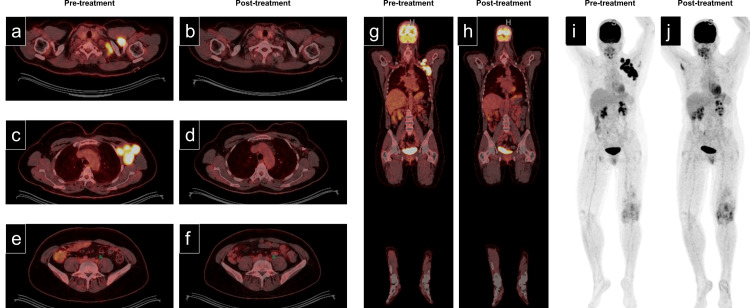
Radiographic positron emission tomography/computed tomography (PET/CT) imaging Positron emission tomography/computed tomography (PET/CT) images. Corresponding pairs of images (a, c, e: pretreatment; b, d, f: posttreatment) were acquired before and after treatment. (a, b) supraclavicular, (c, d) axillary, (e, f) lower abdomen/pelvis. The green arrow in images e and f demonstrates decreased size and fluorodeoxyglucose (FDG) avidity after treatment. No new areas of focal avidity were noted after treatment. (g, h) Pre- and posttreatment coronal views. (i, j) Pre- and posttreatment maximum intensity projection (MIP) images. The focus of the FDG uptake involving the left knee was attributed to pre-existing inflammatory arthritis.
